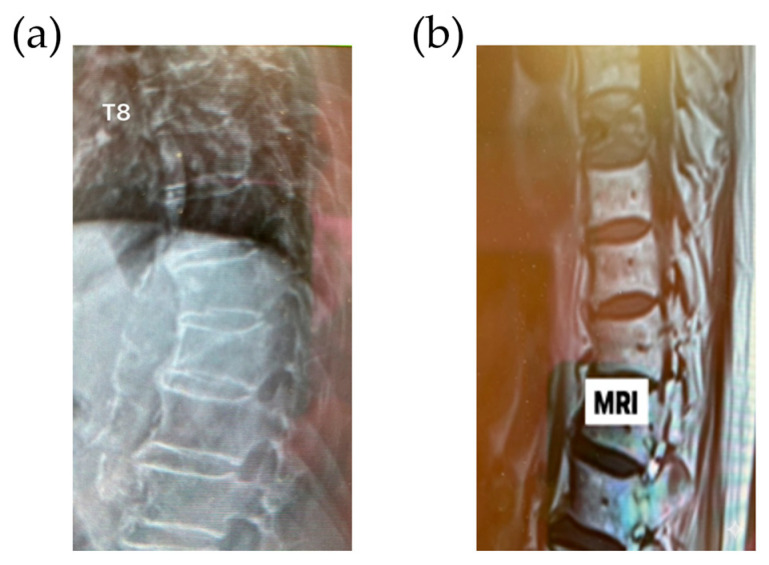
(a) Spine radiograph and (b) T1-weighted sagittal MRI demonstrating a compression fracture at T8 with partial loss of vertebral body height.
